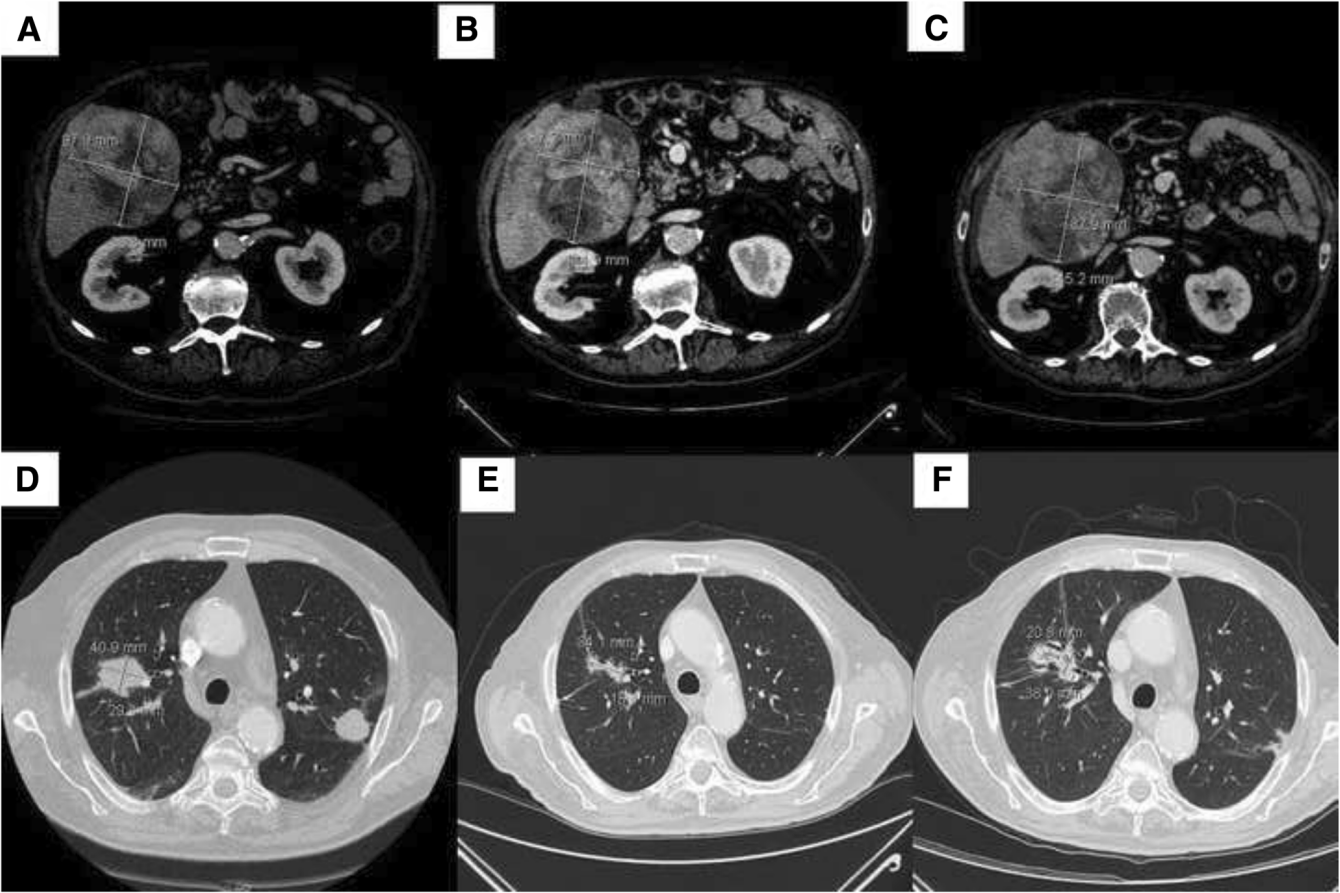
CT scan confirmed the presence of liver, a and lung lesions, d. CT re-evaluation after 6 months showed stability of HCC, b, a partial response in the right lobe of the lung and a complete response in the left lobe of the lung, e. Disease progression in the lung and liver, c-f
